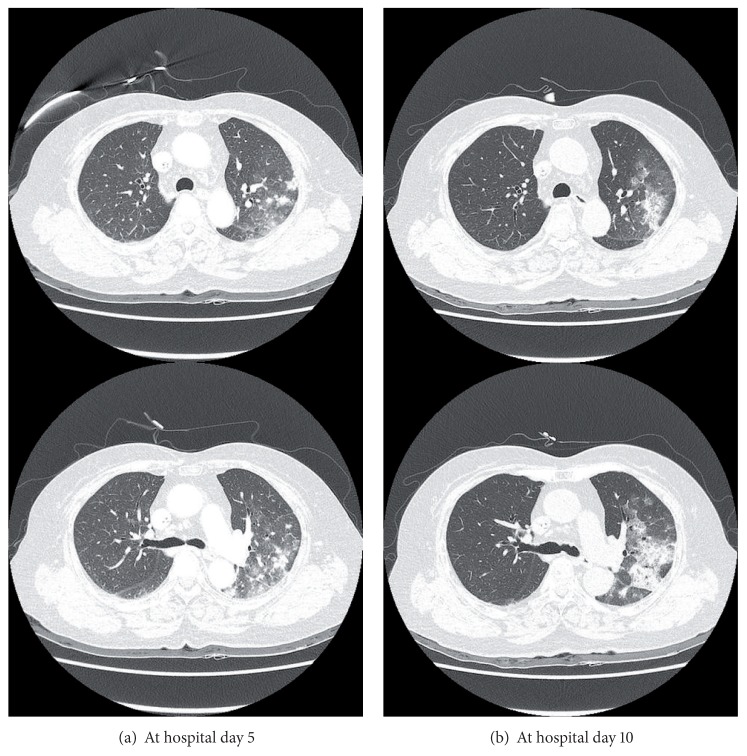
Chest CT findings of lung infiltration.
